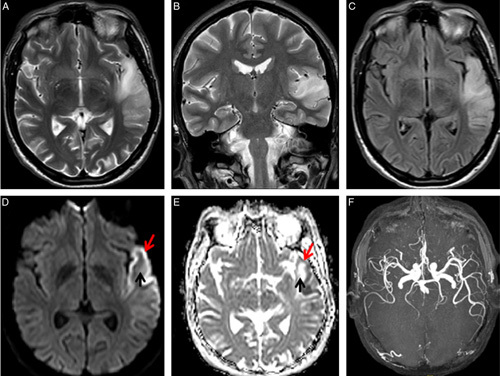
MRI Brain showing T2/FLAIR hyperintensity involving the left superior temporal region and insular cortex showing restriction on diffusion weighted imaging. MR angiogram showing normal flow related enhancement without any occlusion.
